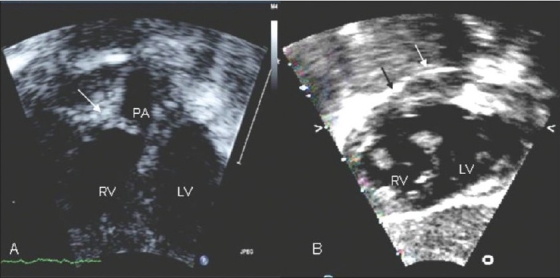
Assessing suitability for BPV by echocardiogram. A: Apical four chamber view with anterior tilt showing predominant valvar PS with doming pulmonary valve (white arrow). B: subcostal short axis view showing predominant infundibular PS (black arrow) with insignificant valvar component (white arrow). RV= right ventricle; LV= left ventricle; A= pulmonary artery
